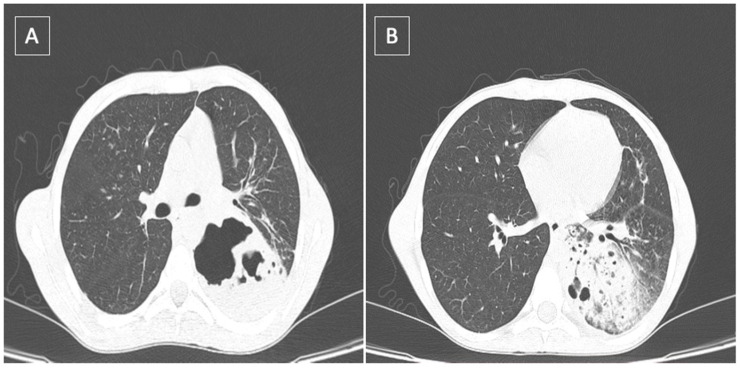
Thoracic CT scan showing: (A) lung abscess located in the left lower lobe; (B) presence of the left lower consolidation with air bronchogram and perilesional ground glass opacities.
